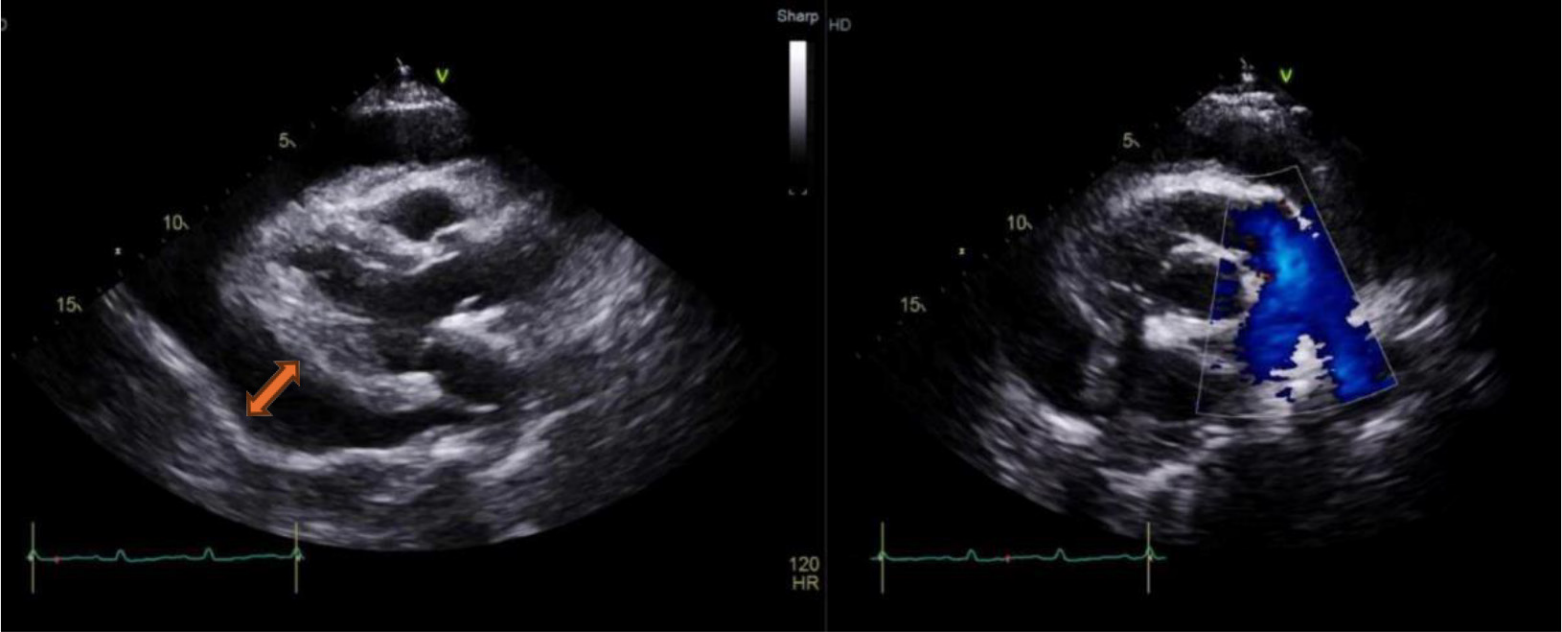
Transthoracic echocardiogram showing a large pericardial effusion (orange arrow) with impending cardiac tamponade.
